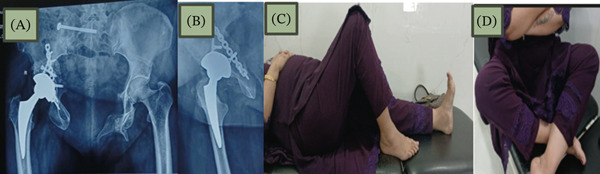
(A, B) Anteroposterior pelvic radiographs at 3‐month follow‐up demonstrating stable osseointegration of the acetabular and femoral components. (C, D) Clinical photographs obtained at 3 months postoperatively showing a good range of motion.
